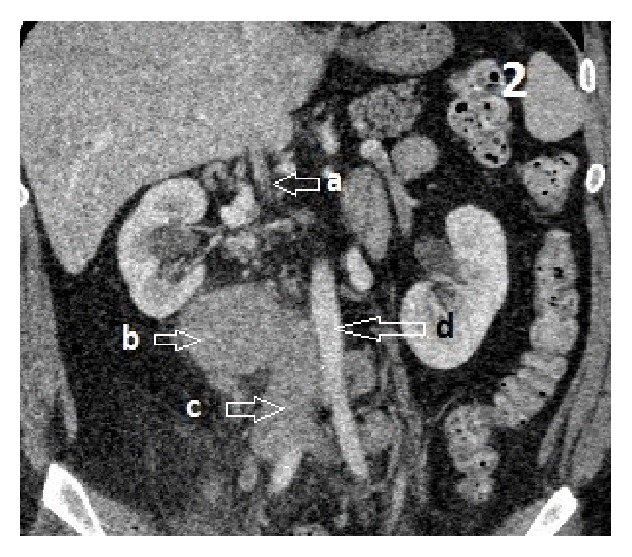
Abdomen CT coronal view: (a) atretic intrahepatic IVC, (b) IVC venous varix, (c) thrombosed IVC, and (d) aorta.
